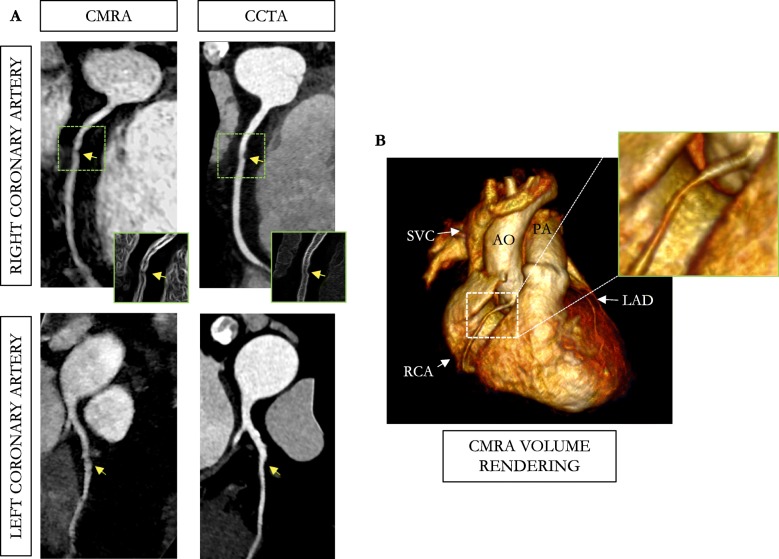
CMRA (0.9 mm^3^ resolution) in a 52-year-old female patient (patient 1) with coronary artery disease. The CMRA data was acquired in 8 min 37 s with 100% scan efficiency at an average heart rate of 58 bpm. a The reformatted CMRA images (left-hand column) disclose a stenosis (arrow) in the mid RCA that matches the lesion observed on the CCTA (right-hand column). A significant narrowing was observed on both modalities with a 50% diameter reduction, compared with normal reference proximal diameter. The same patient showed calcified coronary plaque in the mid LAD (calcium mass: 2.88 mg, Agatston score: 16.1) leading to luminal narrowing of the mid and distal segments of the coronary artery observed on the CMRA and CCTA (arrow). b Volume rendered image of the 3D CMRA dataset is shown. Abbreviations: CMRA, coronary magnetic resonance angiography; CCTA, coronary computed tomography angiography; LAD, left anterior descending artery; RCA, right coronary artery; SVC, superior vena cava; AO, aorta; PA, pulmonary artery
